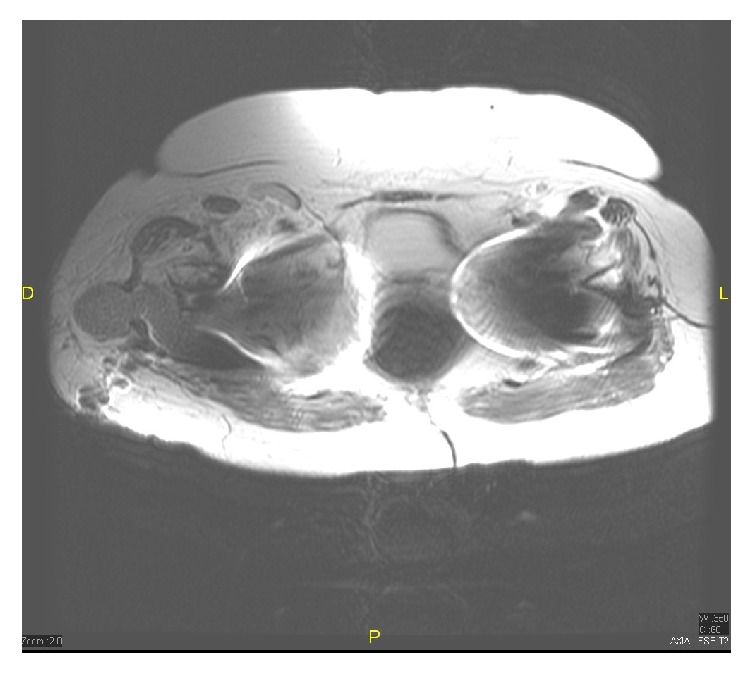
A preoperative right hip MRI. This is a T2 fat-sat weighted MRI axial cut showing the well-organized collection extending into the right prosthesis.
